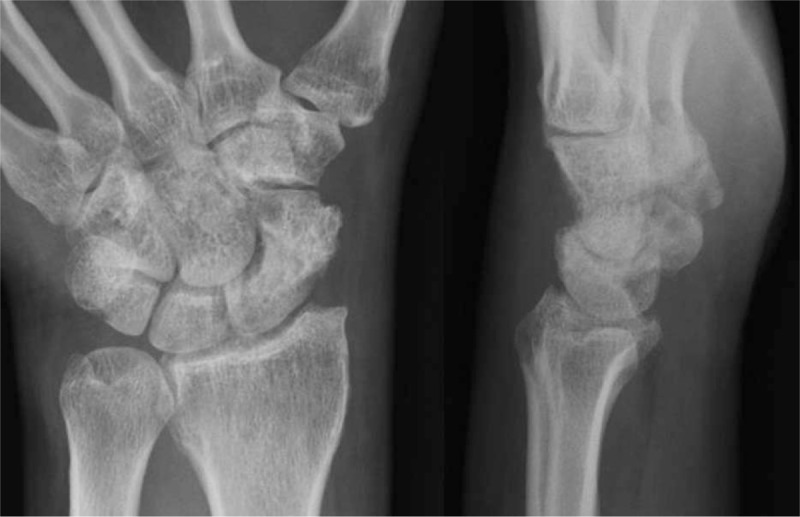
Postoperative 11 weeks K-wires removal plain left wrist plain PA with ulnar deviation and lateral radiographs shows complete bony union. PA = posteroanterior.
